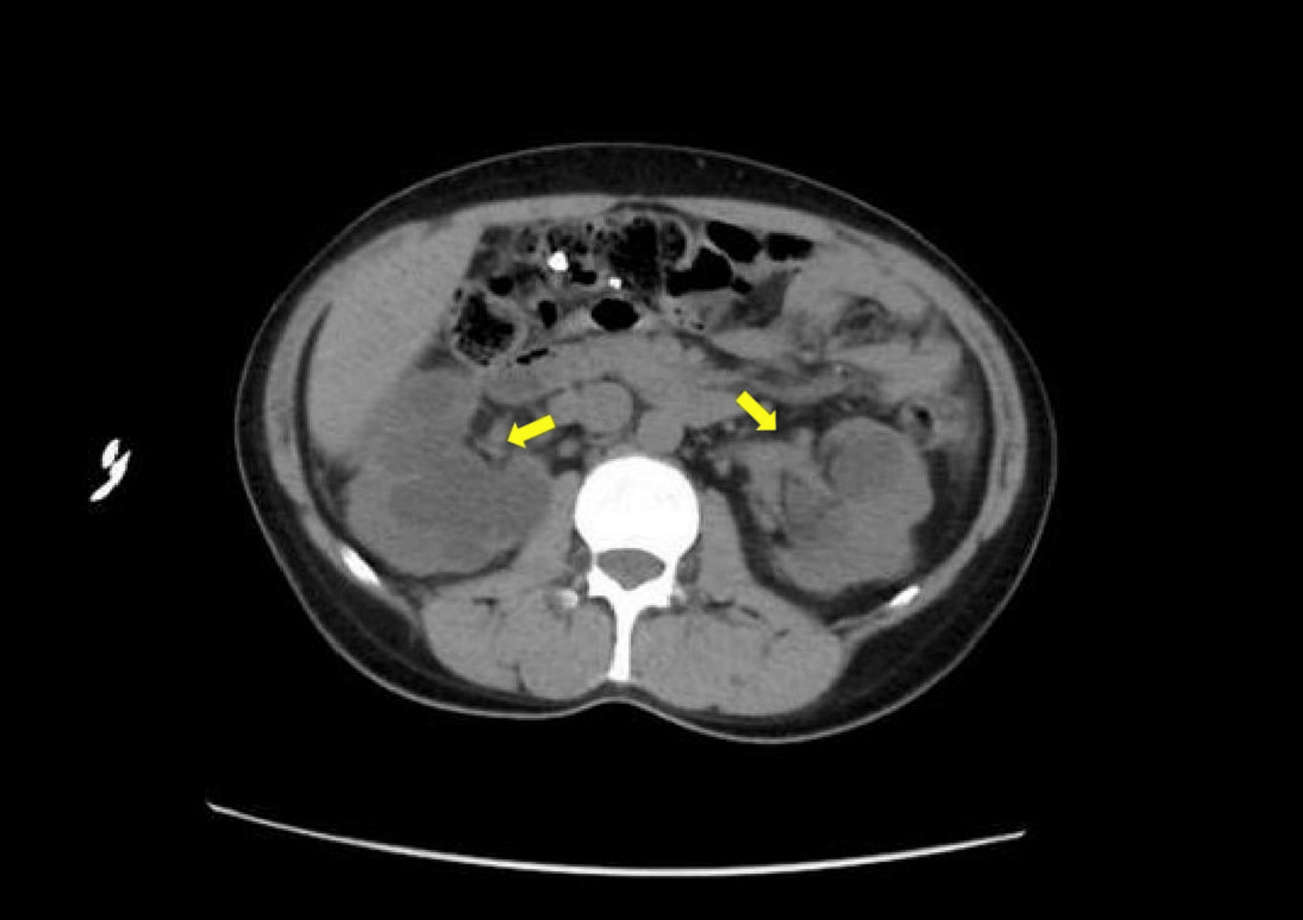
A cross-sectional view of CT abdomen pelvis without IV contrast showing hydronephrosis before stenting.
